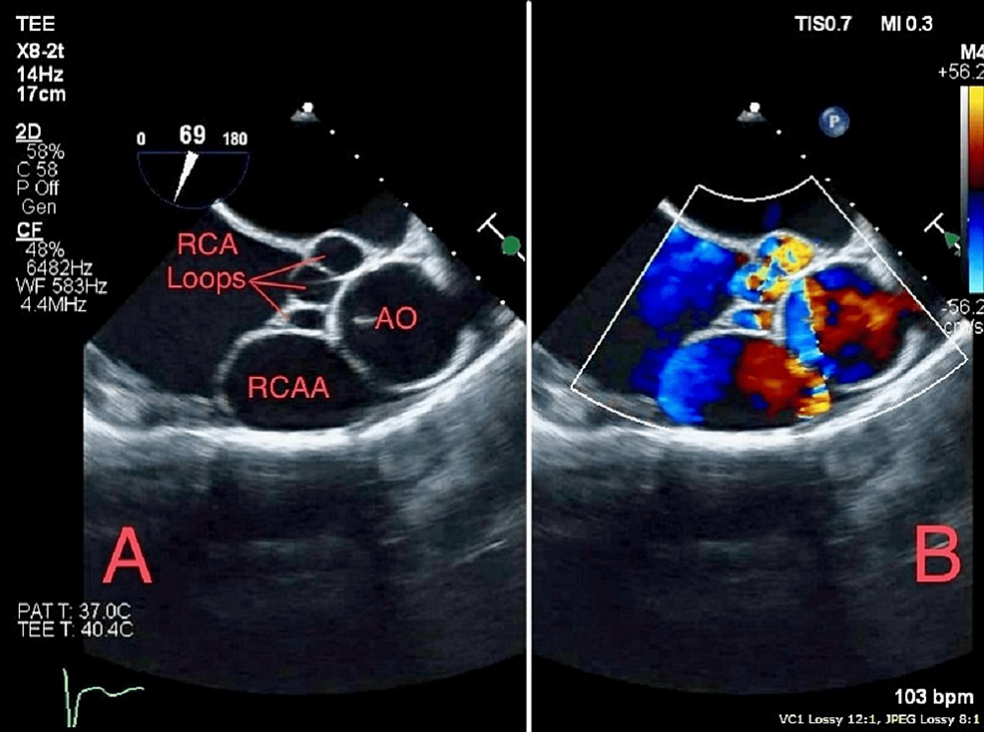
(A) Transesophageal echocardiogram demonstrating RCAA and RCA-RA fistula. (B) Blood flow is represented. Image obtained from the patient’s chart. RCAA: right coronary artery aneurysm; AO: aorta; RCA: right coronary artery
